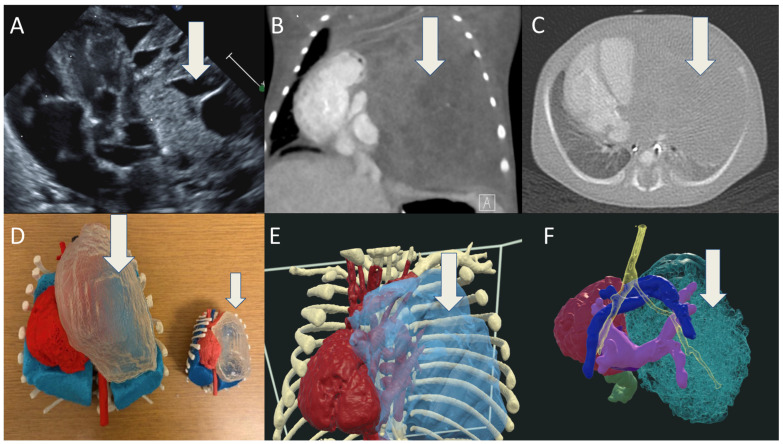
(A) TTE showing the teratoma (arrow) and the heart on the right chest; (B,C) CT chest revealing the multicystic mass in the left chest and displacement of the heart into the right thorax; (D) is a 3D printed model of the teratoma; (E,F) VR segmentation of the teratoma and narrowing of the left bronchus, arrow.
